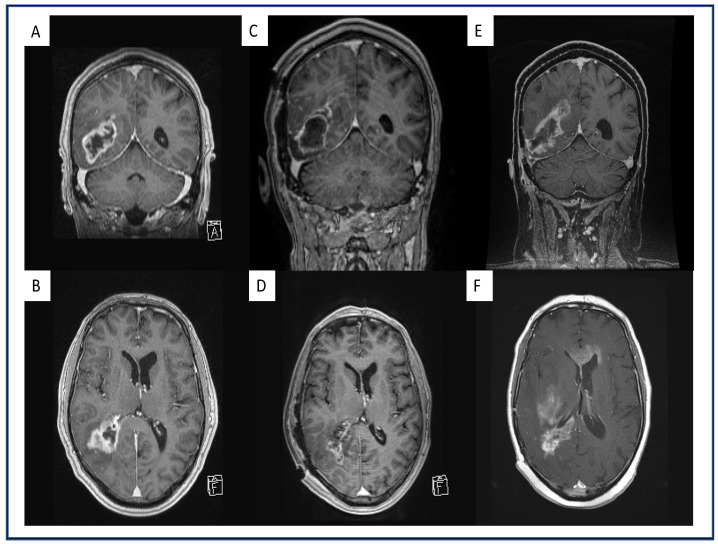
Exemplary representation of a multifocal, midline-crossing GBM under therapeutic AC on the first recurrence after standard-of-care treatment. Preoperative coronal (A) and axial (B) gadolinium-enhanced, T1-weighted MRI of a GBM patient and a right parieto-occipital contrast-enhancing lesion. Postoperative coronal (C) and axial (D) gadolinium-enhanced, T1-weighted MRI of the same patient after GTR where no contrast enhancement is present. Coronal (E) and axial (F) gadolinium-enhanced, T1-weighted MRI of the same patient with therapeutic AC upon first progression with multifocal, midline-crossing GBM recurrence in the right hemisphere.
